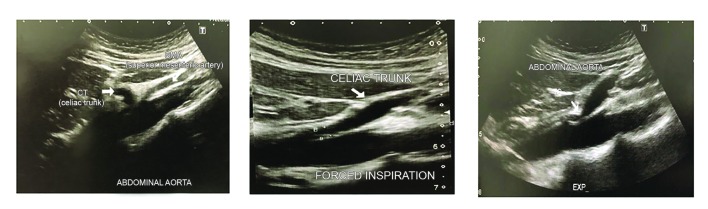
US images showing end-expiratory (right panel) compression of the CT. Left panel, at rest; central panel, with deep inspiration; right panel, with deep expiration. CT = celiac trunk; SMA = superior mesenteric artery; EXP = expiration.
